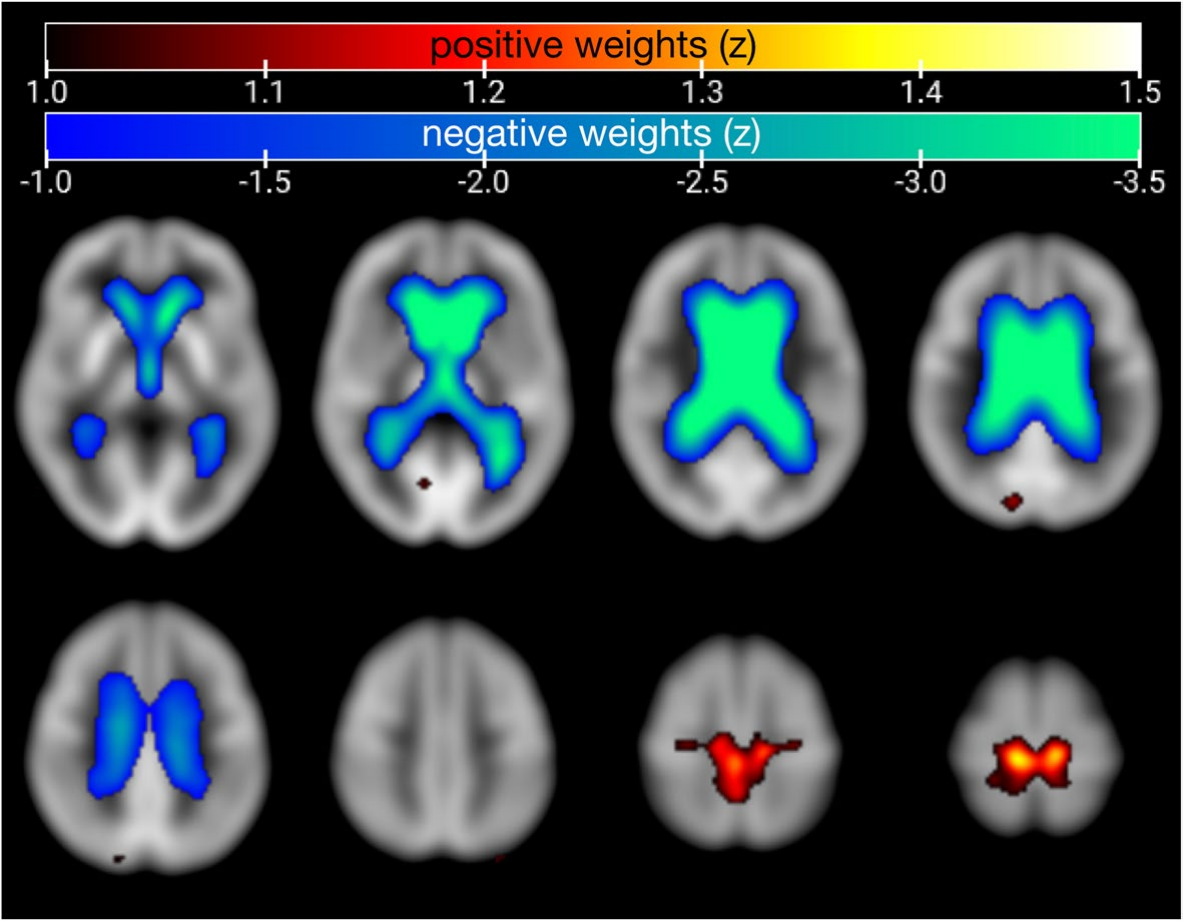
SSM-PCA-based metabolic covariance pattern of iNPH as assessed in patients with definite iNPH (*N* = 11) versus healthy elderly controls (*N* = 48), superimposed on axial slices of an in-house FDG PET template in MNI space. Threshold for visualization purposes at *z* > 1.0 for positive weights and *z* <  − 1.0 for negative weights. Image depicted in radiological orientation, i.e., the left image side corresponds to the patient’s right body side
